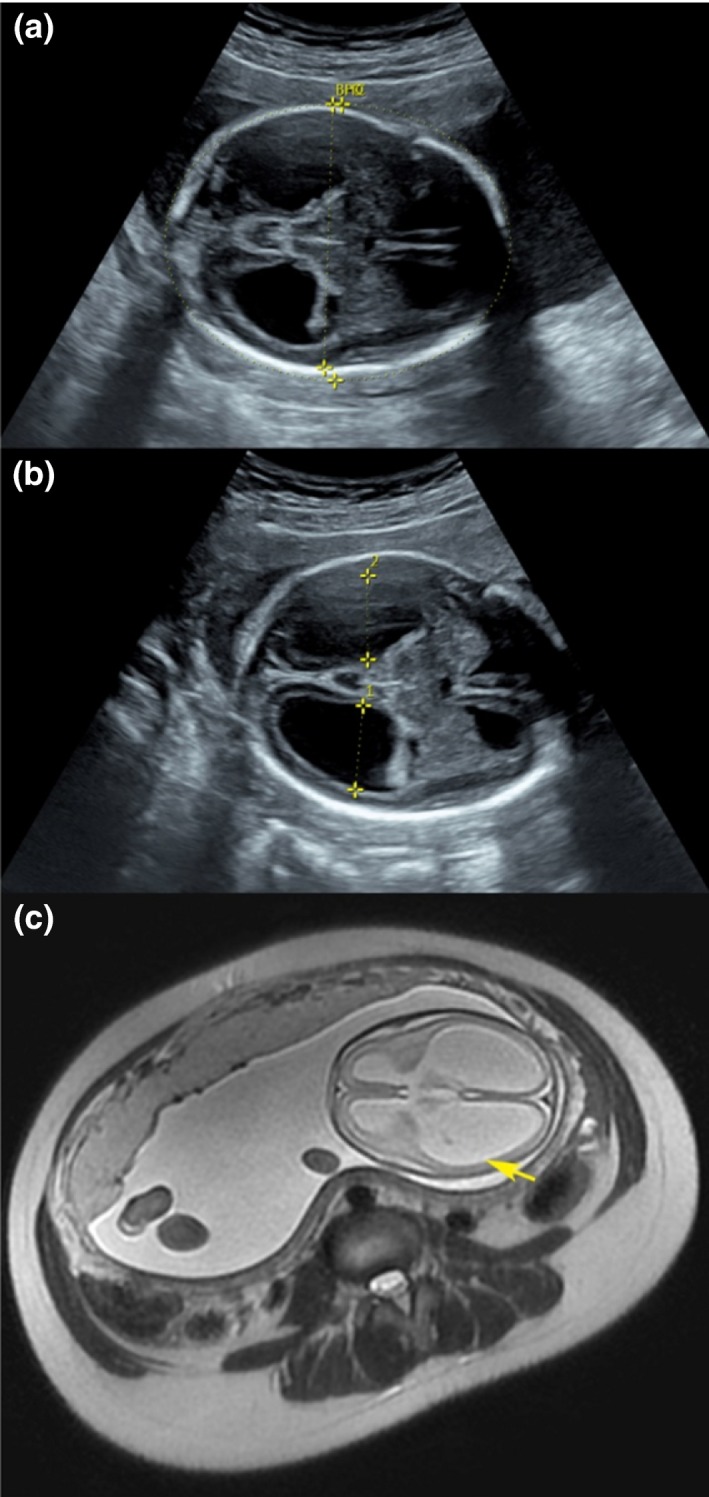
The ultrasound scan and magnetic resonance imaging (MRI) scan. (a) The ultrasound scan, BPD = 6.54 cm, HC = 24.36 cm; (b) The ultrasound scan, 1D = 2.09 cm, 2D = 2.08 cm; (c) MRI scan
